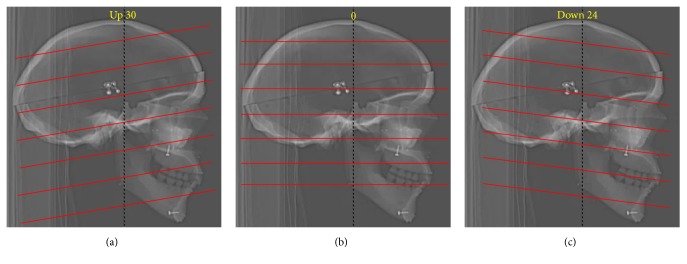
The CT machine was tilted from superior 30° to inferior 24° in 6° increments, yielding 10 CT scans with different tilts for 3D reconstruction. The red lines in the figures indicate the angles at which the CT X-ray beams irradiated the model after tilting. The images indicate the CT scan X-ray beam irradiation angles for (a) superior 30° tilt, (b) 0° tilt, and (c) inferior 24° tilt.
